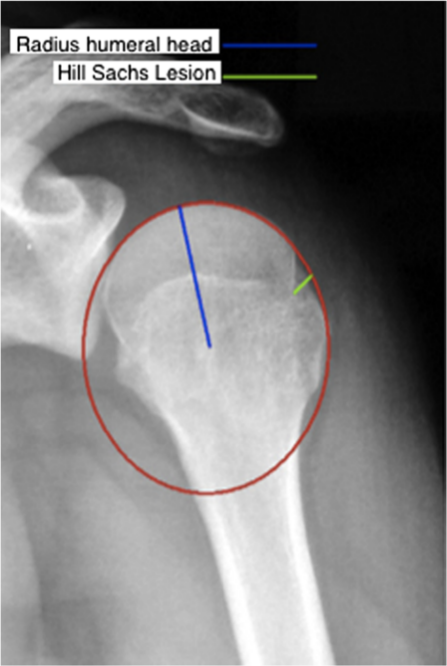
Humeral head depth:radius ratio (d/R). On a true AP x-ray with internal rotation, a circle template is fit to the contour of the articular surface of the humeral head and the depth of Hill-Sachs bone loss is measured. (Adapted from Sommaire *et al.* [46])
